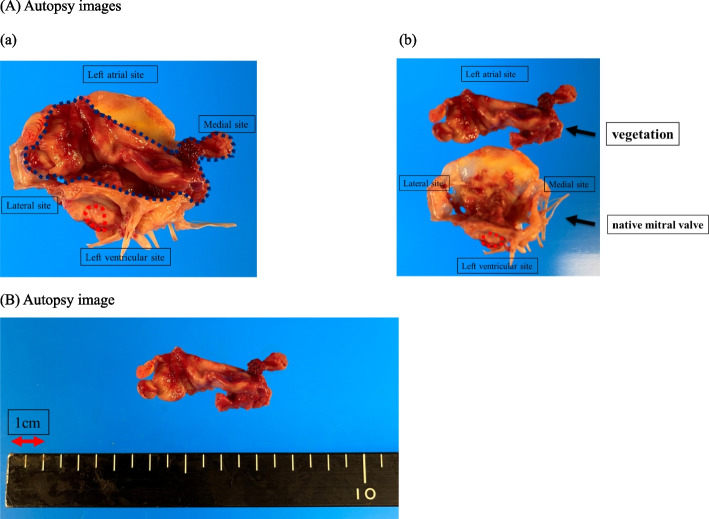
The autopsy findings were similar to the findings of transthoracic echocardiography, showing a large vegetation on the left atrial side. A Dorsal view of the vegetation and mitral valve. a Image of the giant vegetation attached to the mitral valve. b Image of the mitral valve and the giant vegetation isolated from the mitral valve. B The size of the giant vegetation was measured to be about 5 cm in width and 1.5 cm in length. Red dotted line: MitraClip®. Grey dotted line: vegetation
